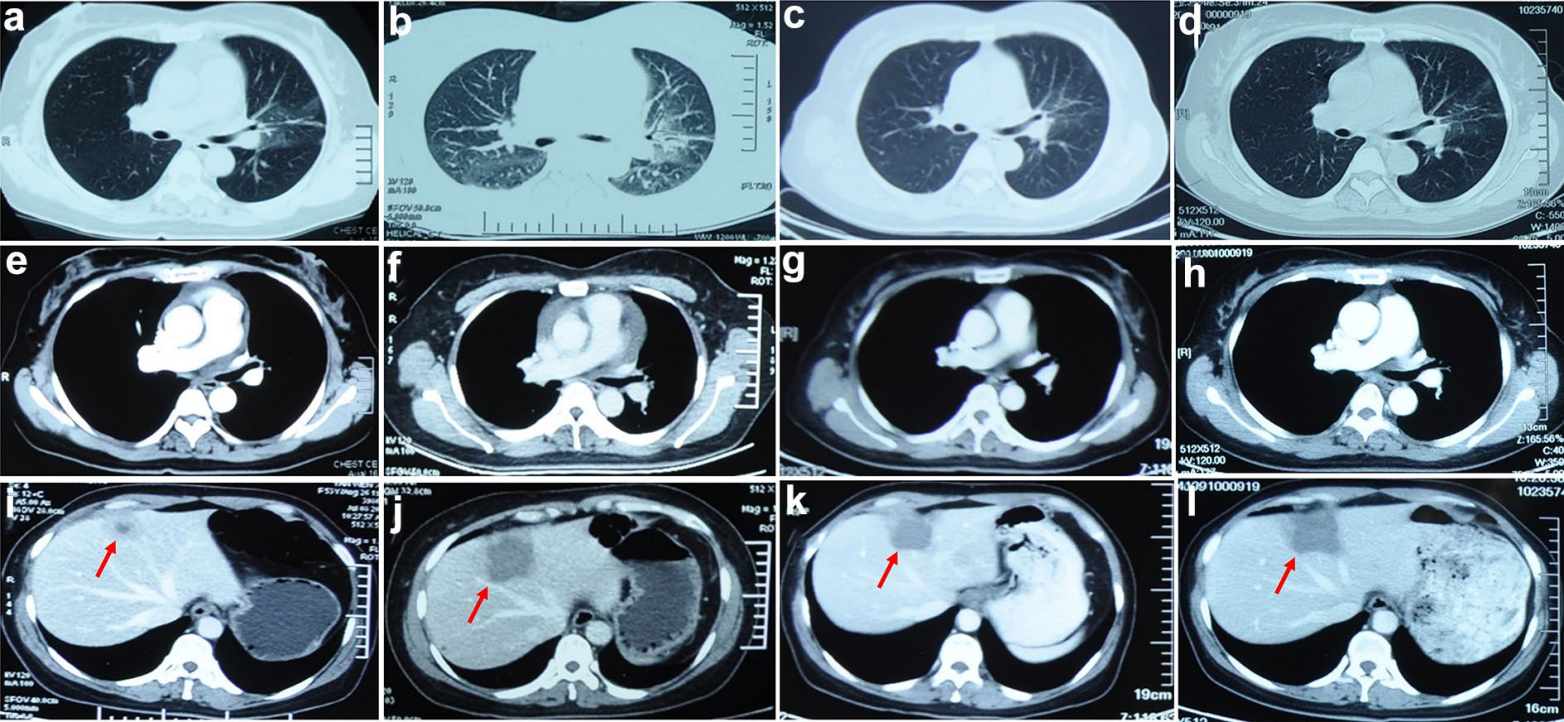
Disease evaluation during the treatment of crizotinib and ceritinib. a–h The CT scan revealed complete remission of lung lesions. i A single liver nodule was found after the 6 months of starting crizotinib. j The enlarged liver lesions was revealed on 20 Aug. 2014. k The liver metastasis shrinked after the treatment of ceritinib. l The liver lesions enlarged again after the 5 months of starting ceritinib
